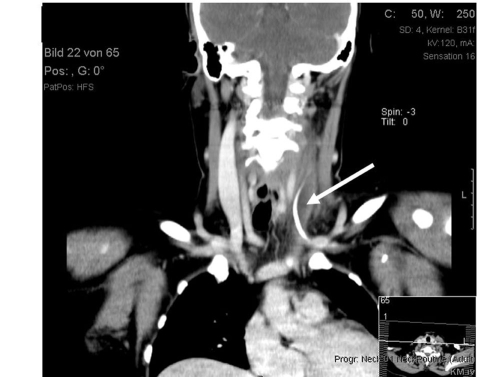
CT-scan representing thrombosis of the left internal jugular vein due to a dislocated left subclavian catheter (white arrows).
